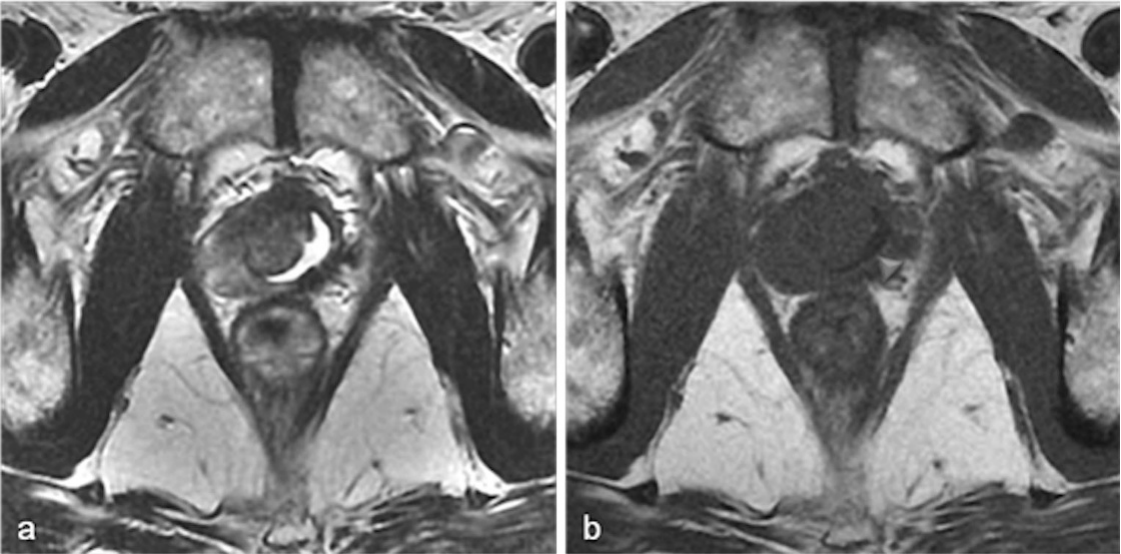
Residual fluid cavity. Six-month post-treatment follow-up MRI in a patient with left photodynamic therapy. Axial T2-weighted (a) and T1-weighted (b) MR images show an irregularly shaped residual fluid cavity in the treated lobe, with a low signal on T1 (*arrowhead*) and a high signal on T2
